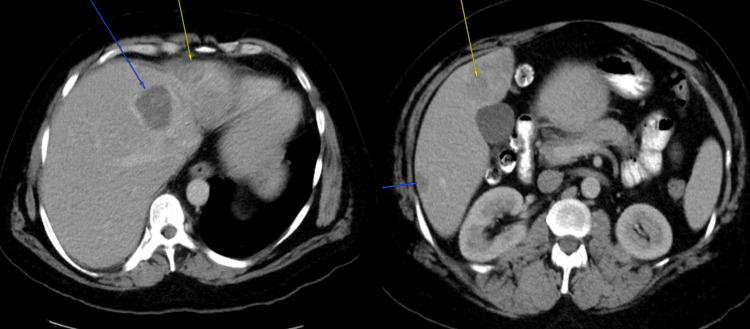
Numerous lesions, indicated by blue and yellow arrows, measuring between 2.2 and 6.4 cm, were observed in various parts of the liver during an abdominal computed tomography (CT) scan.
